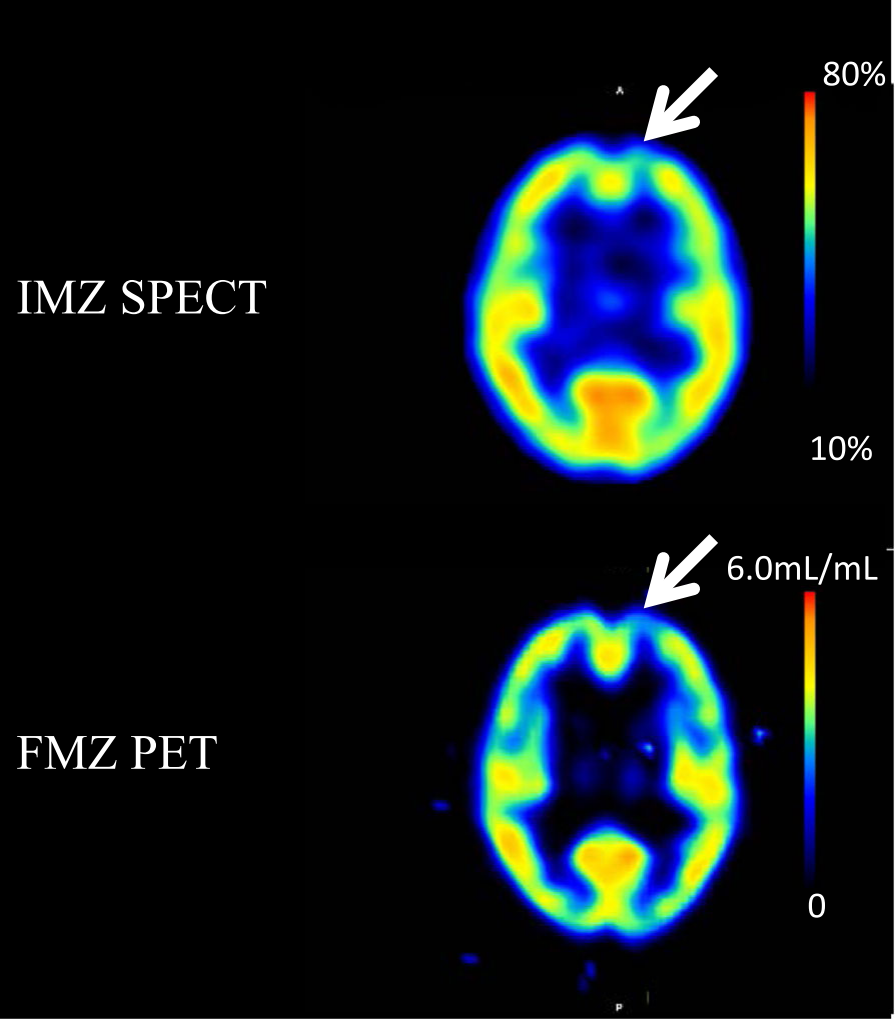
Case 2 (Patient No. 7): The patient was a 29-year-old female who was diagnosed as having acute subdural hemorrhage at the time of injury and complained of mental fatigue and headache after the injury. FMZ BP_ND_ was low inside the left frontal lobe. The low uptake level inside the left frontal lobe was also observed by IMZ SPECT
